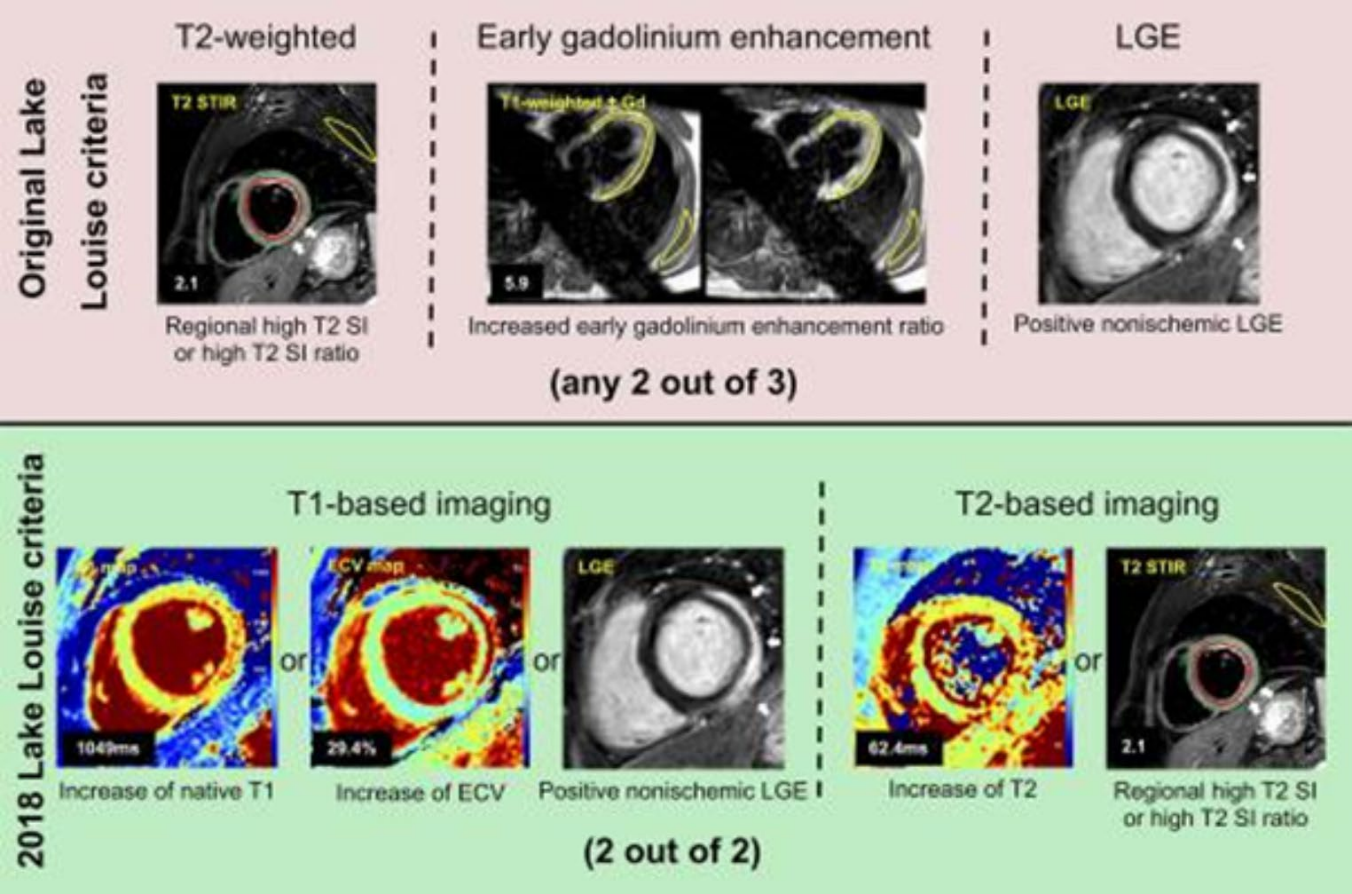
Original and 2018 Lake Louise criteria (LLC) in a 24-year-old man with acute myocarditis. Original LLC consisted of three main criteria: regional high T2 signal intensities on T2-weighted images (white arrows) or increased global T2 signal intensity ratio, increased early gadolinium enhancement ratio on T1-weighted images, and areas with high signal intensities in nonischemic distribution pattern on late gadolinium enhancement (LGE) images (white arrows). 2018 LLC consist of two main criteria (T1-based criterion and T2-based criterion). T1-based criterion is considered to be positive if increase of native T1 relaxation times, increase of extracellular volume (ECV), or positive LGE (white arrows) exist. T2-based criterion is positive in cases of increased T2 relaxation times or in cases with regional high T2 signal intensities on T2-weighted images (white arrows) or increased global T2 signal intensity ratio. Gd = gadolinium, SI = signal intensity, STIR = short tau inversion recovery.Reprinted from [12]. No changes were made
